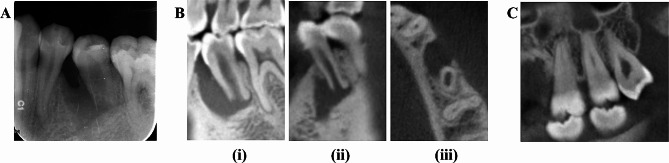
(A) The preoperative IOPA radiography of tooth #35, depicting thin dentine walls and an open apex, with extensive periapical radiolucency. (B) The preoperative CBCT images from sagittal (i), coronal (ii) and horizontal (iii) planes, further revealed the extent of the bony defect and the large diameter of the immature apex. (C) CBCT information of the third molar tooth #28 in the sagittal view, showing no dentinal and periapical radiolucency
